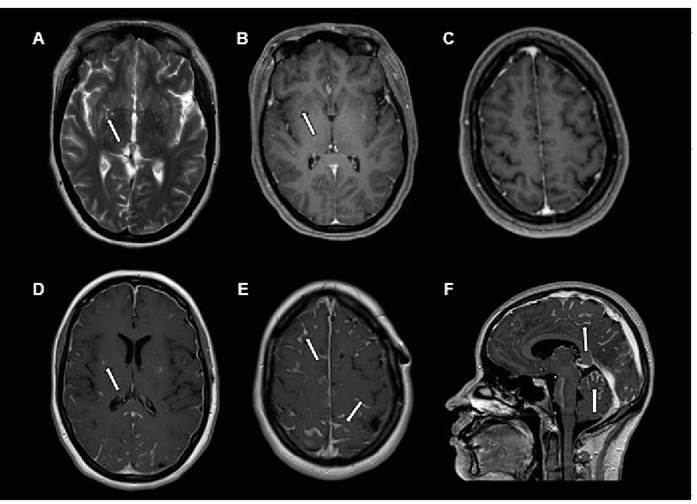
Magnetic resonance imaging axial T2-weighted image showing enlargement of the perivascular spaces in the nucleocapsular regions (arrows) (A). Axial contrast-enhanced T1-weighted image showing minimal enhancement in the enlarged perivascular spaces (arrows) (B). Axial contrast-enhanced T1 image on high convexity, with no enhancement of leptomeningitis (C). Axial post-contrast T1-weighted image showing persistent enhancement of the enlarged perivascular spaces; note the marked leptomeningeal enhancement that is more conspicuous in the bilateral parieto-occipital region (D and E). Sagittal post-contrast-enhanced T1-weighted image showing marked leptomeningeal enhancement in the frontal, parietal, and occipital lobes in the supratentorial region and also between the cerebellar folia in the infratentorial region, with the presence of leptomeningitis (F).
